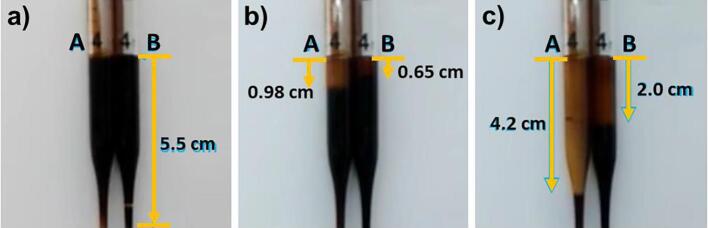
Viscosity measurements of (A) raw and (B) ultrasonicated (100 W for 30 min) lacquer sap solutions: a) ready to drop, b) after 1 s, and c) 2 s.
